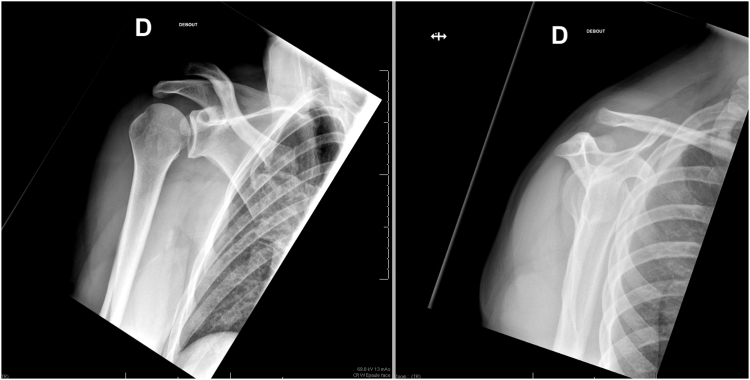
Preoperative right shoulder X-ray (anterior-posterior and Neer views, showing stage IV acromioclavicular dislocation).
